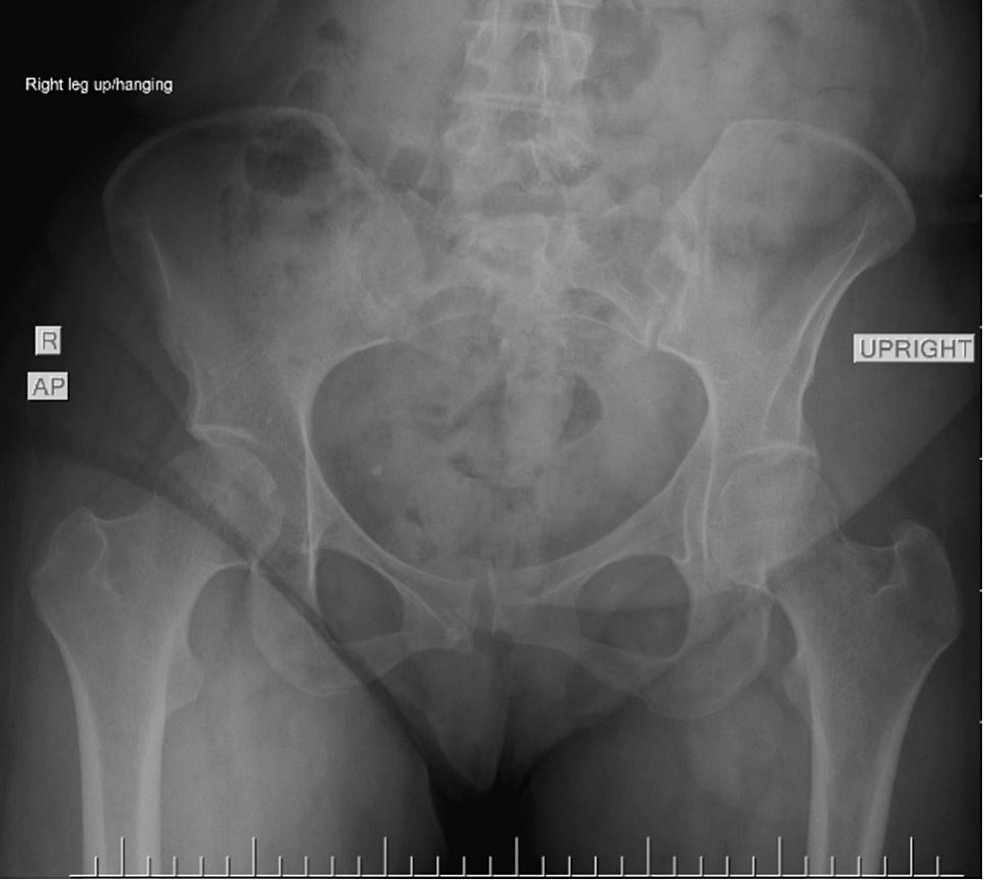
Anteroposterior (AP) radiographic view of the pelvis as a stress view, with the right leg elevated, demonstrating pubic symphyseal widening along with widening of the patient's left sacroiliac (SI) joint, defined as pelvic ring instability
